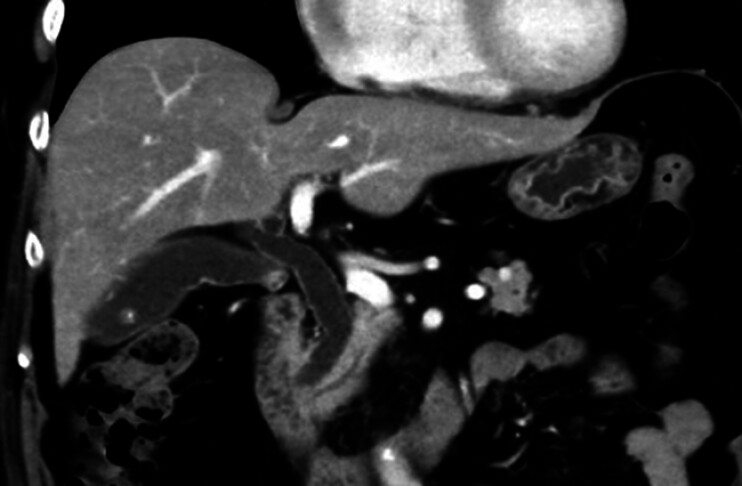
Contrast-enhanced computed tomography showed common bile duct dilation and mild wall thickening.
